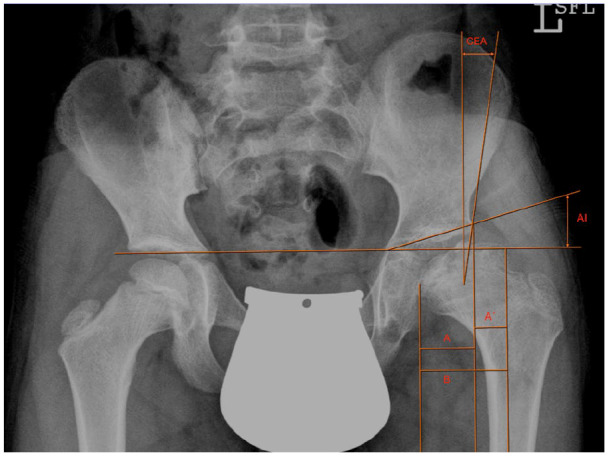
Preoperative radiograph and radiographic measurements of a 9-year-old boy with severe LCPD of the left hip with inadequate femoral head coverage and hip joint incongruence. AI, acetabular index; CEA, centre-edge angle; FHC, femoral head coverage percentage = A/B × 100; RI, Reimer’s index = A’/B × 100. AI, acetabular index; CEA, centre-edge angle; FHC, femoral head coverage percentage = A/B × 100; RI, Reimer’s index = A’/B × 100.
